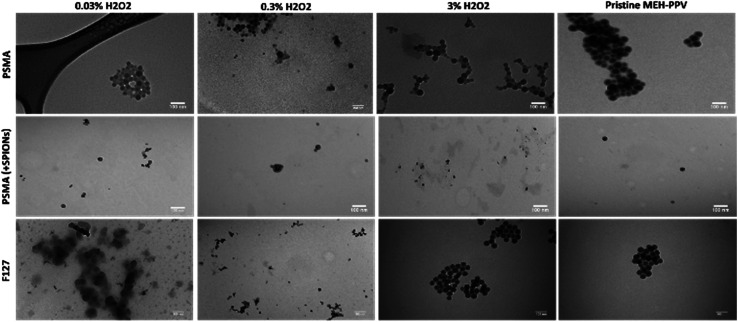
TEM images of the oxidised MEH-PPV, Taken at 100× resolution (scale bar = 100 nm). Top line is oxidised MEH-PPV:PSMA NPs, middle line is oxidised MEH-PPV:PSMA:SPIONs, and bottom line is oxidised MEH-PPV:F127.
